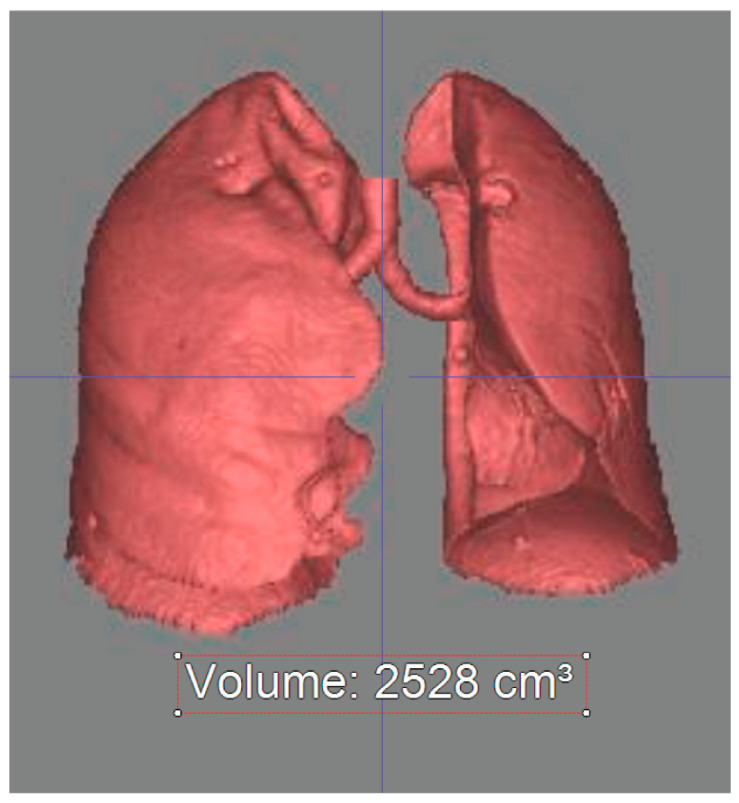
Three dimensional lung volume measurement on chest CT.
